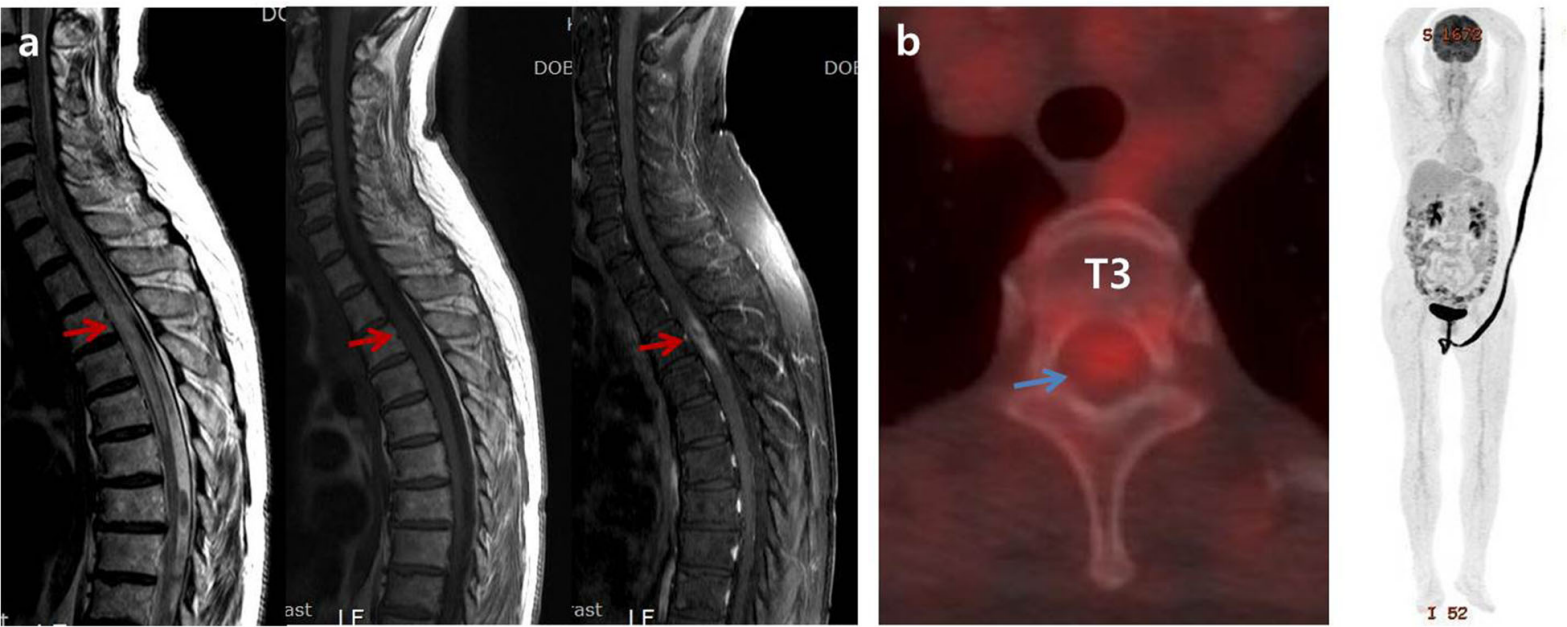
Preoperative magnetic resonance imaging shows intramedullary mass lesion with iso-intense on T2-weighted images (WI) and iso- to slightly low-intense on T1-WI on T2-4, which was heterogeneous enhanced pattern with cord swelling and edema from C7 to T6 (red arrows) (a). There was a single lesion on T2-4 with maximum standardized uptake value (SUV_max_) of 3.14 and no systemic lesion on whole-body ^18^F-fluorodeoxyglucose positron emission tomography/computed tomography (blue arrow) (b)
